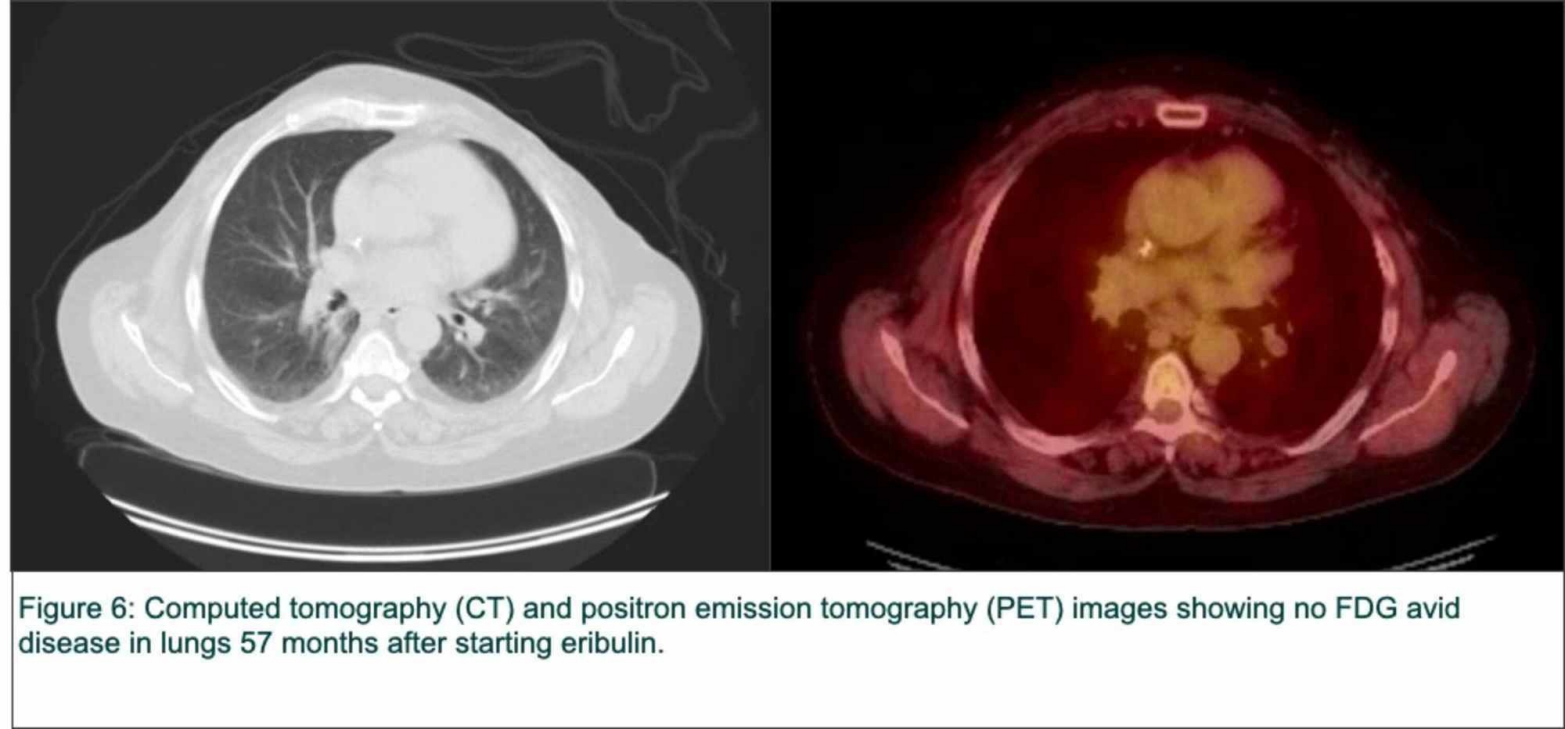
Computed tomography (CT) and positron emission tomography (PET) images of the lungs 57 months after starting eribulin FDG: fluorodeoxyglucose.
